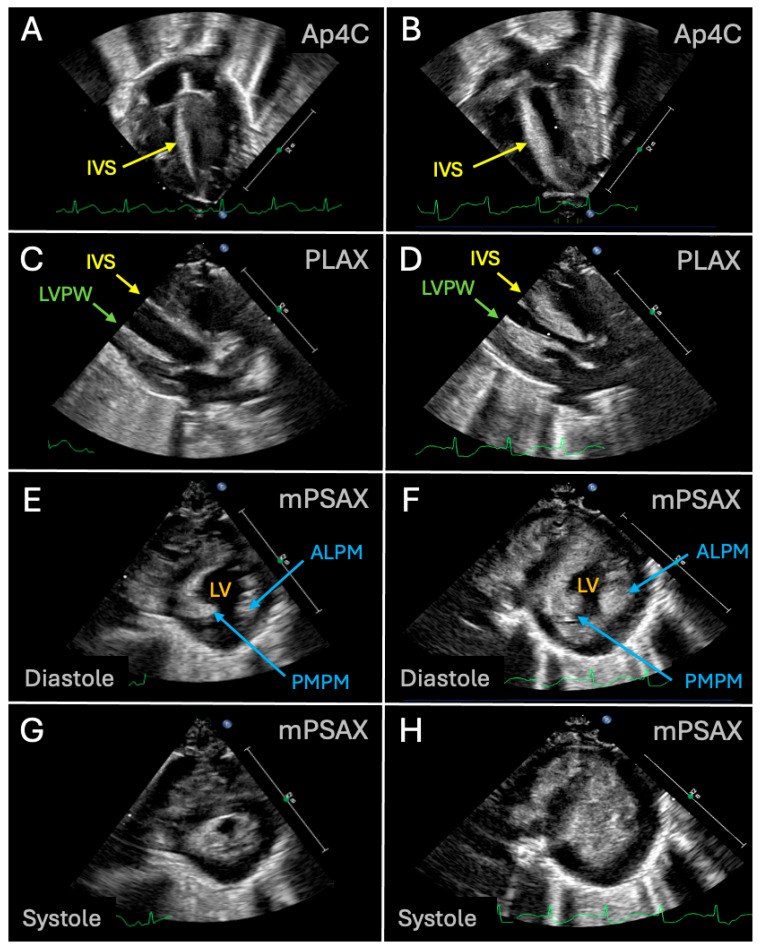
Conventional 2D echocardiographic images of a neonatal heart (left: (A,C,E,G)) and one with reactive hypertrophic cardiomyopathy (right: (B,D,F,H)). Of note: (D) LV obstruction due to concomitant reactive hypertrophy of the IVS and LVPW and (F,H) uniform, concentric reactive LV hypertrophy as shown on the mPSAX view. Abbreviations: Ap4C (apical four-chamber view); IVS (interventricular septum); PLAX (parasternal long-axis view); LVPW (left ventricular posterior wall); ALPM (anterolateral papillary muscle); PMPM (posteromedial papillary muscle); mPSAX (modified parasternal short-axis view).
